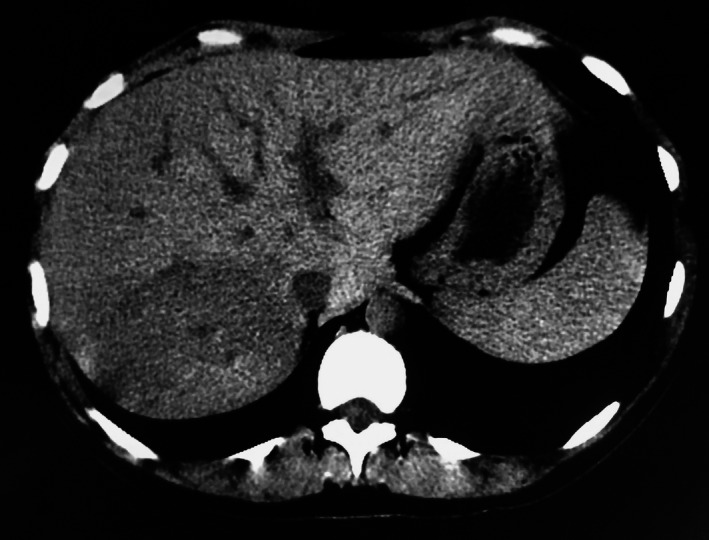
CT after delivery : showed a well‐limited zone corresponding to the US hepatic nodule.
